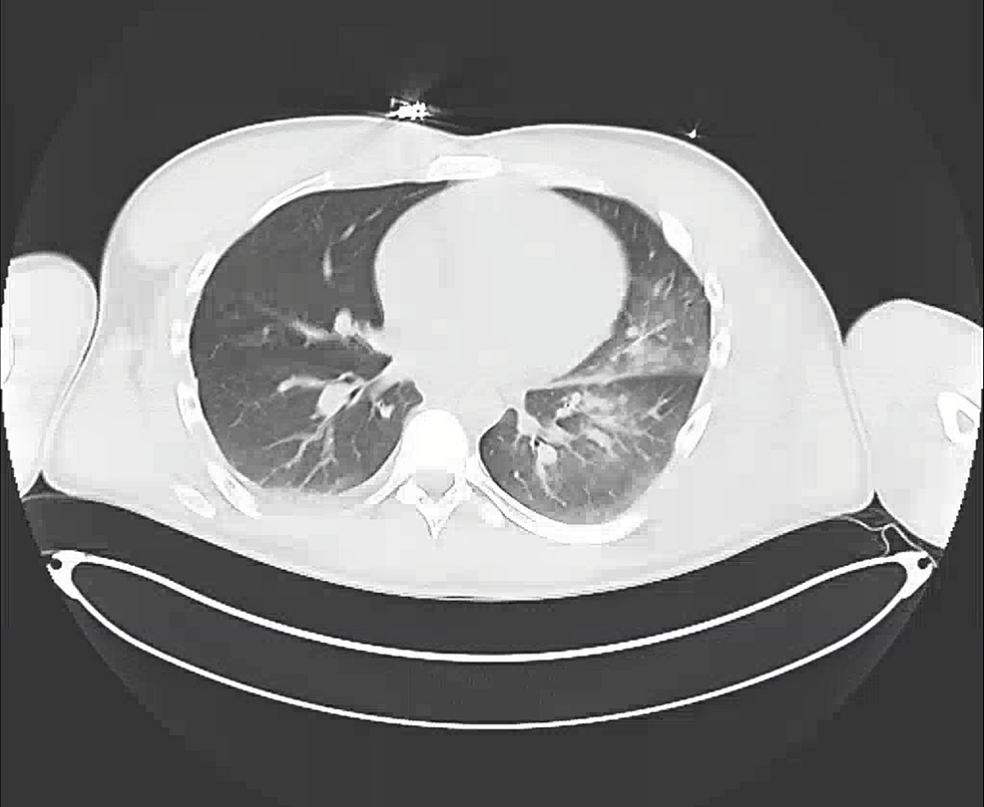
CT of the chest showing bilateral aspiration pneumonia.
